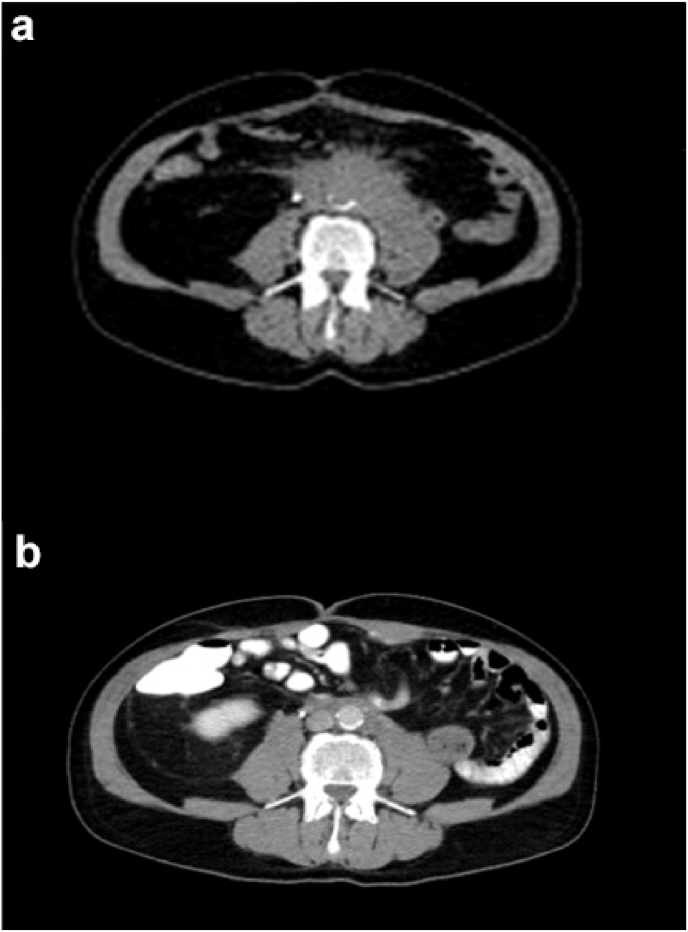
CT images before (a) and after (b) introducing steroid treatment with significant decrease in size of the retroperitoneal mass.
